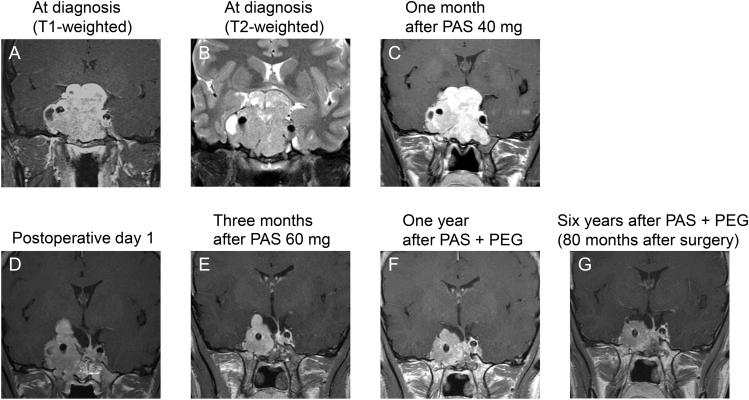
Serial pituitary MRI during the clinical course. (A) Coronal contrast-enhanced T1-weighted MRI at diagnosis showing a giant PitNET measuring 48 × 48 × 58 mm with suprasellar extension and bilateral cavernous sinus invasion (Knosp grade 4). (B) Coronal T2-weighted MRI demonstrating high signal intensity. (C) MRI after 1 month of preoperative pasireotide (PAS) therapy, showing modest tumor reduction (48 × 46 × 51 mm). (D) Early postoperative MRI demonstrating residual tumor (28 × 46 × 51 mm) invading the cavernous sinus. (E) MRI 3 months after PAS 60 mg, showing further reduction (25 × 36 × 51 mm). (F) MRI 1 year after PAS–pegvisomant (PEG) combination therapy, showing stable tumor size (26 × 31 × 48 mm). (G) MRI 6 years after PAS + PEG (80 months after surgery) demonstrating long-term tumor stability (26 × 29 × 42 mm).
